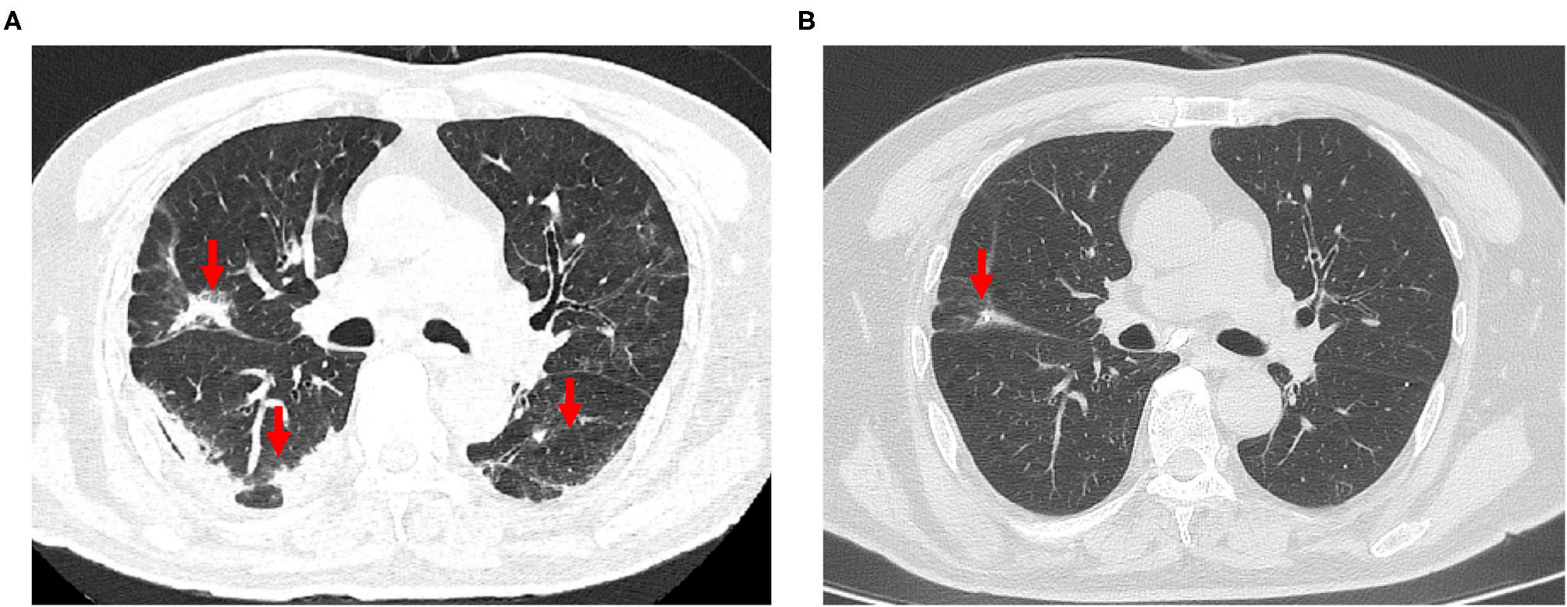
(A) The chest CT scan of a 79-year-old man with severe COVID-19 on admission, revealing diffuse ground-glass opacity and consolidation in the lungs. (B) Reexamination of chest CT at 10 months after discharge, showing that the ground glass opacities and consolidation had completely disappeared, and mild pulmonary fibrosis were present.
